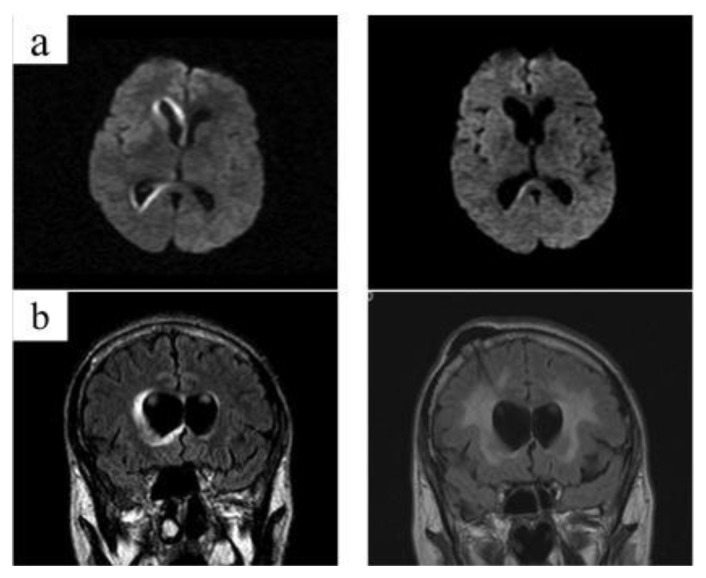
Cranial magnetic resonance imaging (MRI) findings prior to (left column) and after (right column) rituximab-containing chemotherapy. At presentation, MRI diffusion sequence (a) and coronal T2-FLAIR sequence (b) show hyperintense lesions in the periventricular withe matter (predominantly around right lateral ventricle). Follow-up MRI after completion of 2 courses of immunochemotherapy demonstrates marked regression of hyper-intensities (right column). Note Ommaya reservoir placed in right lateral ventricle (b, right column).
